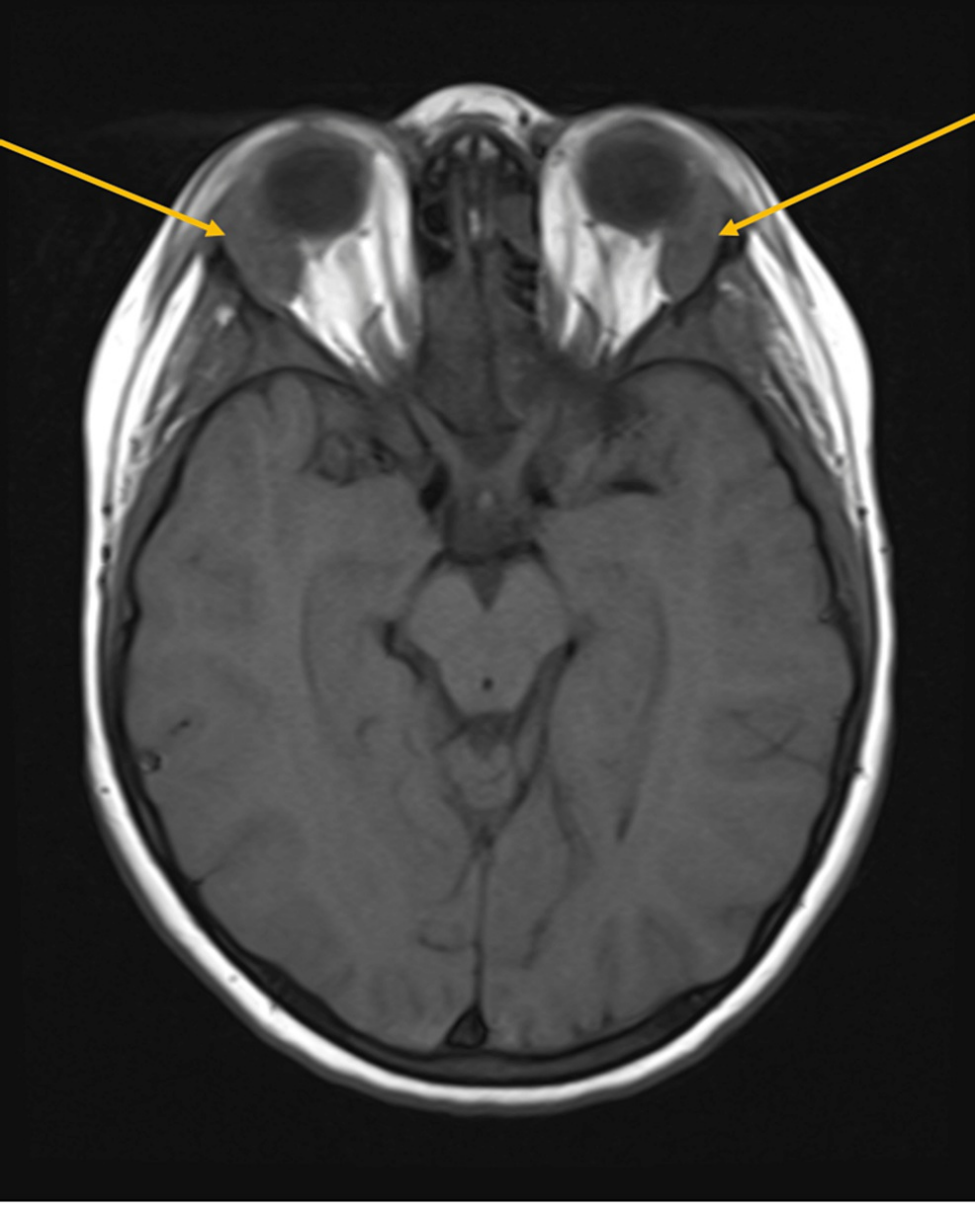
Axial T1-weighted MRI demonstrating intermediate signal of the orbital masses
